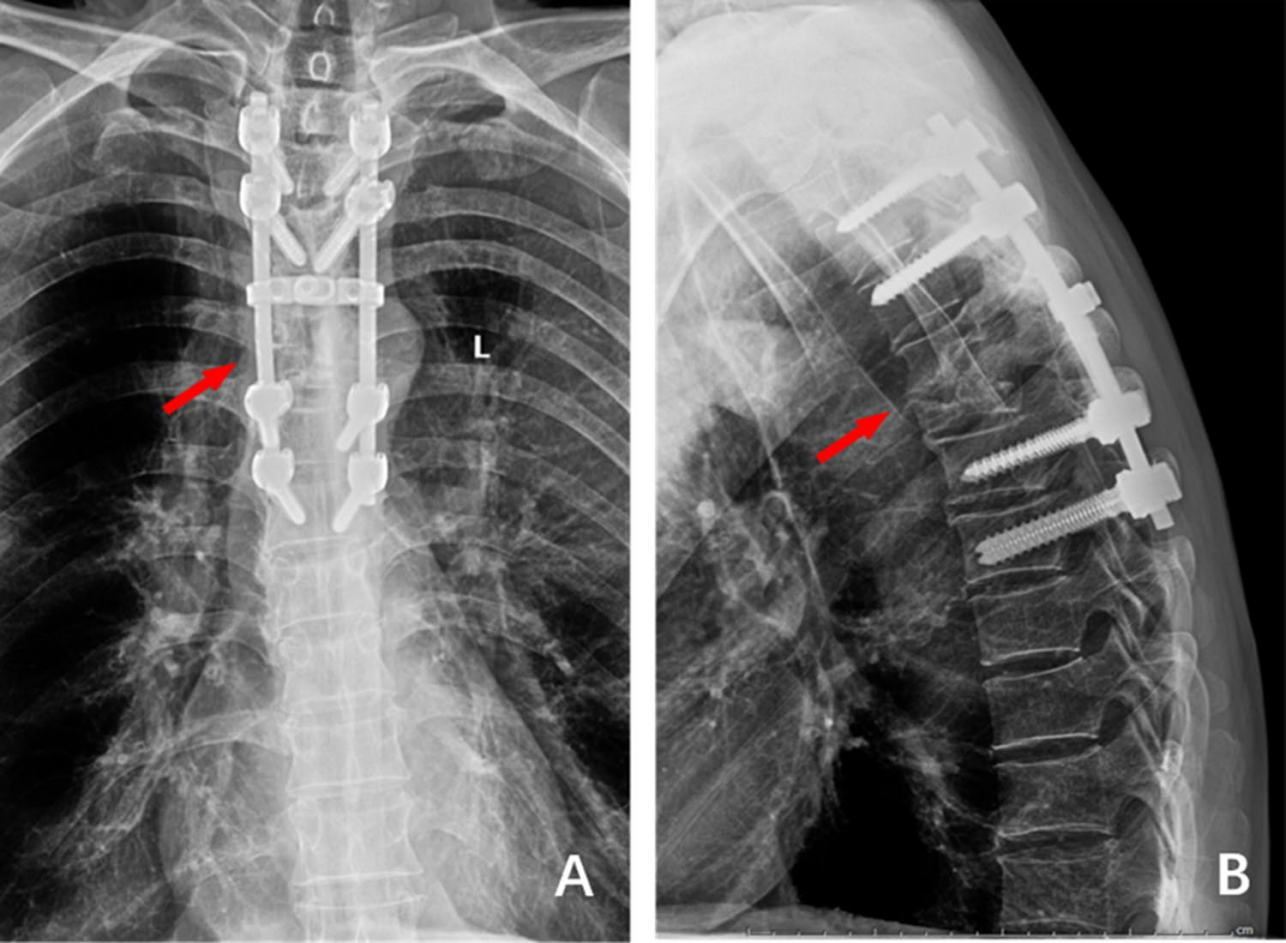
(A) and (B) 1 week after the operation, the frontal and lateral X-ray films showed that the internal fixation position was good, the deformity was corrected well, and The Cobb Angle was 19°.
